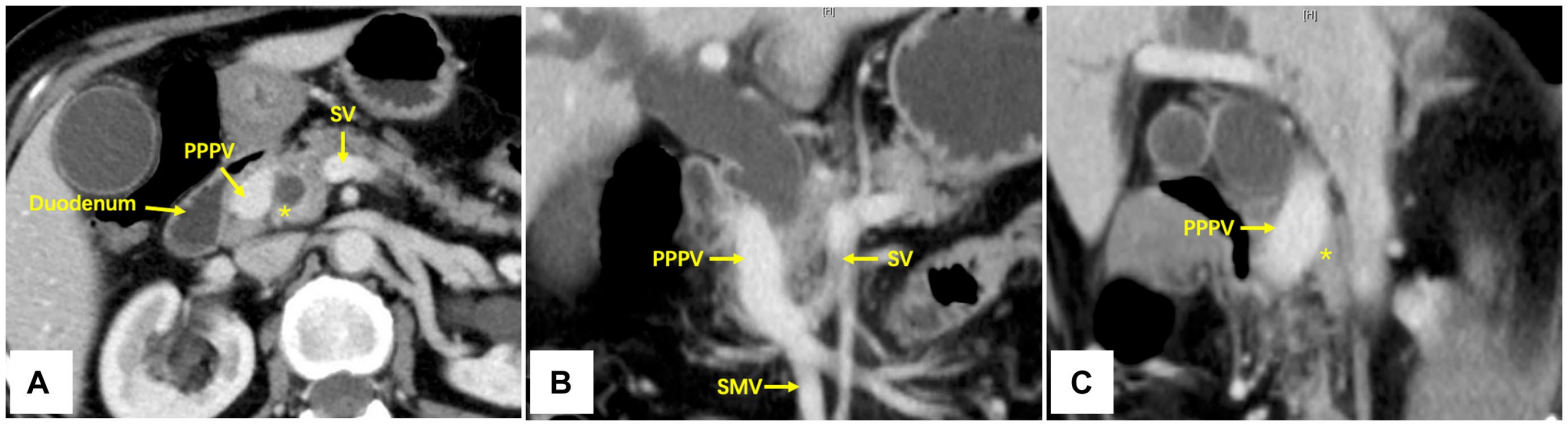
Axial (A), coronal (B), and sagittal (C) imaging of contrast-enhanced CT scan. The PPPV was located anterior to the pancreas and posterior to the duodenum, and the L-shaped SV was longitudinally located posterior to the pancreatic neck. PPPV, prepancreatic postduodenal portal vein; SV, spleen vein; SMV, superior mesenteric vein; *The pancreas posterior to PPPV.
